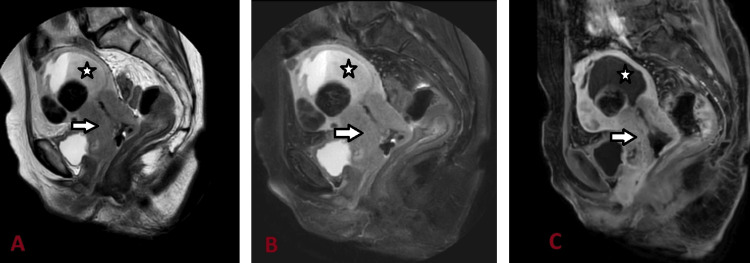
MRI abdomen and pelvis T2 (A), T2-FS (B), and T1-C+ sagittal sections showing heterogeneously hyperintense cervical growth (arrow) involving the lower half of the uterus and the upper half of the vagina with heterogeneous enhancement (C) and resultant hyperintense endometrial collection showing fluid-fluid level (star) and multiple fibroids with calcific degeneration. FS: Fat saturated; C+: Contrast
